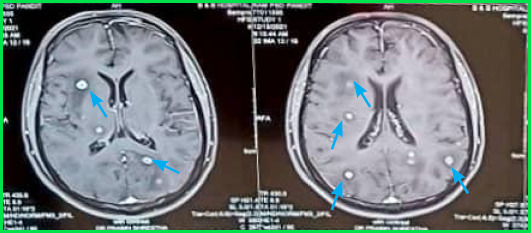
MRI brain (T1, coronal view) showing multiple ring-enhancing lesions.
